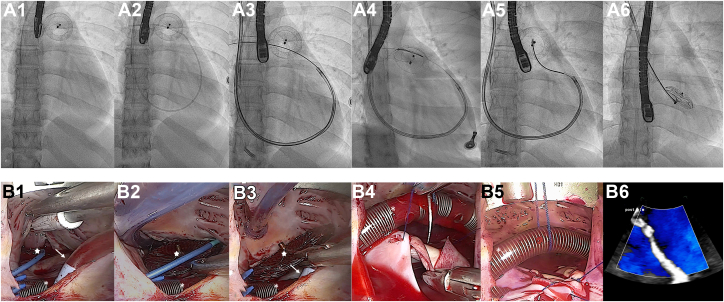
Hybrid Transcatheter-Minimally Invasive Endoscopic Retrieval of an Embolized Atrial Septal Defect Occluder (A1) Baseline fluoroscopy showing the 26-mm Amplatzer atrial septal defect (ASD) occluder (Abbott Structural Heart) projecting over the main pulmonary artery (PA). (A2) Engagement of the right PA with a 5-F angled pigtail catheter introduced through an 11-F right internal jugular sheath. (A3) Advancement of an Amplatz Super Stiff J-tip guidewire (Boston Scientific) through the pigtail catheter, enabling exchange to an 8.5-F steerable guiding sheath (Oscor). (A4) Introduction of a 7-F 3D EnSnare system (Merit Medical) via a 7-F multipurpose catheter through the steerable sheath. Initial snaring of one device pin was successful, but in-sheath slenderizing failed, and the occluder re-embolized to the PA bifurcation (not shown). (A5) Repeat snaring was achieved; however, coaxial alignment and slenderizing remained unsuccessful. (A6) The snared device was mobilized en bloc with the multipurpose catheter and steerable sheath and intentionally parked in the right ventricle. (B1) After establishing cardiopulmonary bypass via percutaneous femoral cannulation with a dual-stage venous cannula (white asterisk), minimally invasive endoscopic access was obtained through a right lateral microthoracotomy. Following cardioplegic arrest and right atriotomy, the parked occluder (white star) is visualized through the tricuspid valve. Left ventricular venting is performed via the ASD using a sump catheter (white arrow). (B2) Release of the EnSnare from the occluder pin (white star) and freeing of the device from the tricuspid subvalvular apparatus. (B3) Endoscopic extraction of the embolized device. (B4) Exposure of the ASD facilitated by anterior suspension of the venous cannula, followed by surgical patch repair. (B5) Endoscopic view after completion of the ASD patch repair. (B6) Transesophageal echocardiography confirming complete closure without residual shunting on color Doppler.
